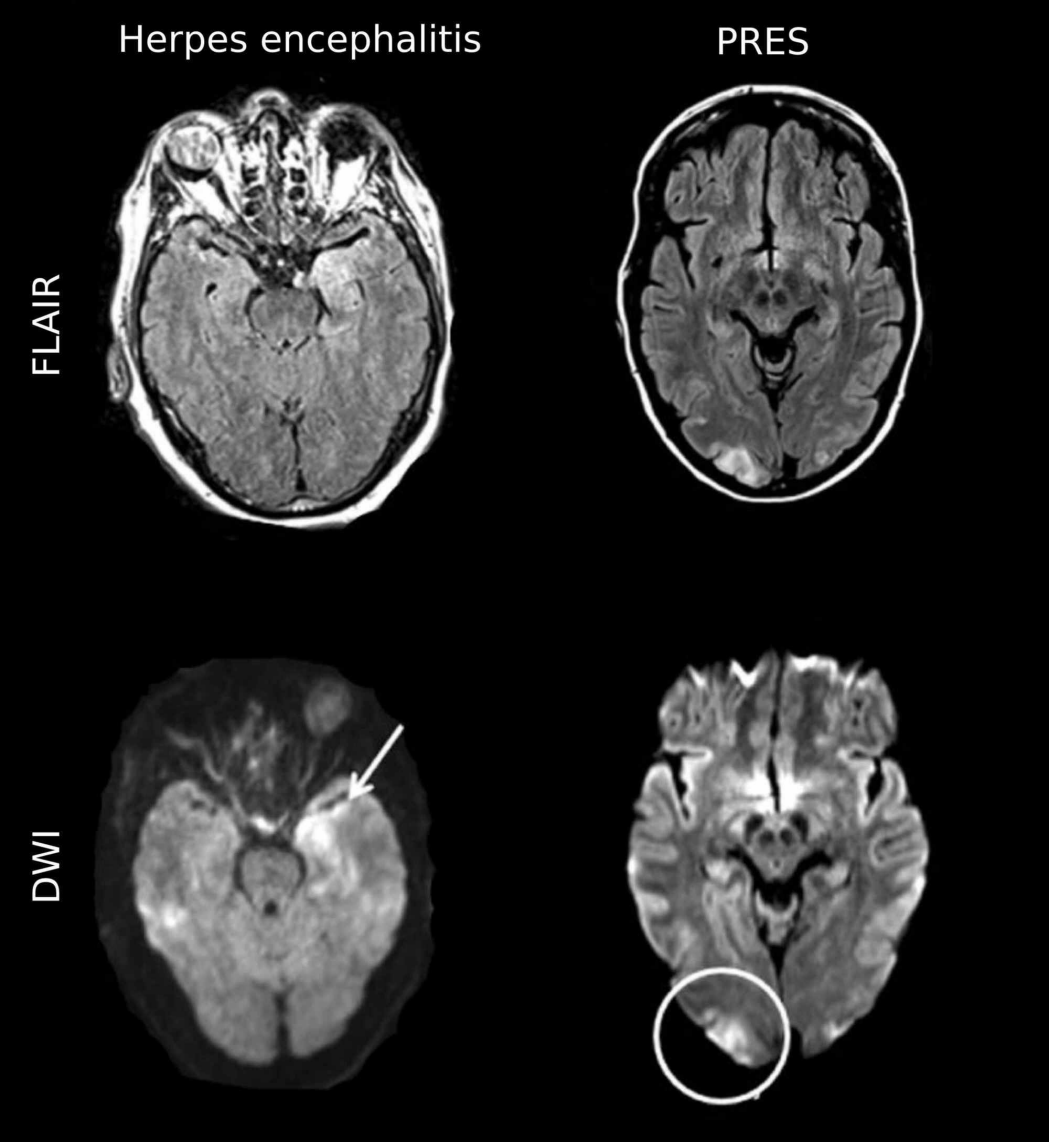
Imaging findings in MELAS differential diagnoses Exemplary FLAIR and DWI images of diseases that can mimic MELAS lesions on MRI, including herpes simplex encephalitis and PRES, are displayed. Herpes simplex encephalitis may mimic many MELAS MRI findings including cortical restricted diffusion (arrow), subcortical vasogenic edema, and local mass effect on FLAIR images. Nevertheless, herpes simplex encephalitis lesions usually affect mesiotemporal areas and spread continuously. PRES lesions are typically located in the occipital and temporal lobes, showing subcortical vasogenic edema (circle) [8]. FLAIR, fluid-attenuated inversion recovery; DWI, diffusion-weighted imaging; MELAS, mitochondrial encephalomyopathy with lactic acidosis and stroke-like episodes; MRI, magnetic resonance imaging; PRES, posterior reversible encephalopathy syndrome
